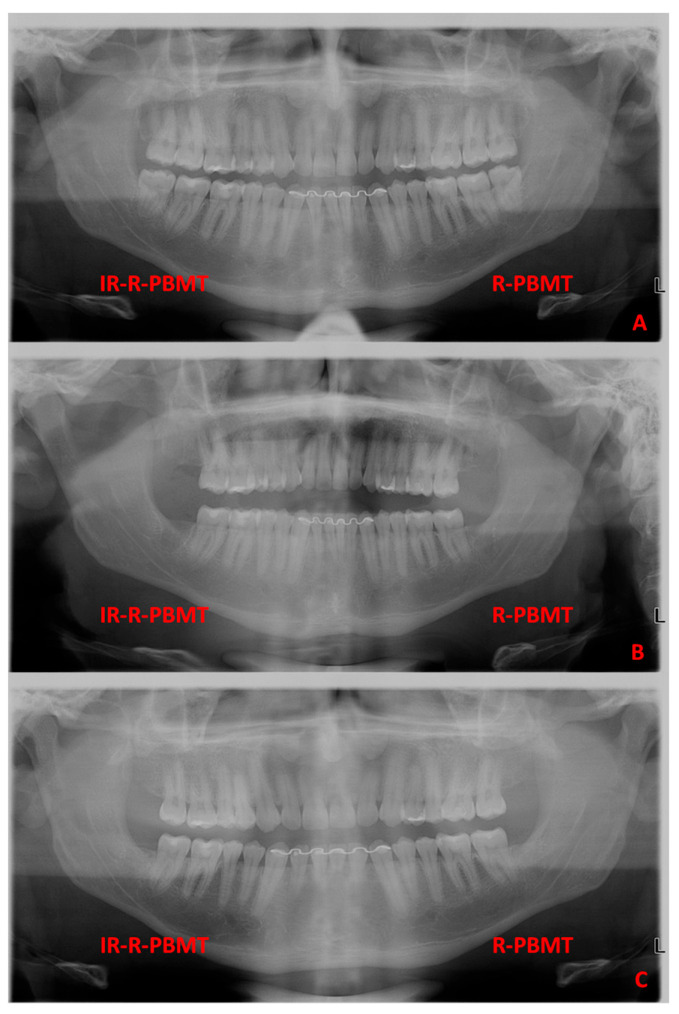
Representative panoramic radiographic images of a patient enrolled in this study. (A) Pre-surgical condition of the third molars; (B) radiographical aspect of the extraction sockets after 7 days of the surgical procedure; (C) the radiographical aspect of the extraction sockets after 90 days of the surgical procedure. It was not possible to detect differences in radiographical density and fractal dimension in the post-extraction sockets treated with IR-R-PBMT and R-PBMT.
